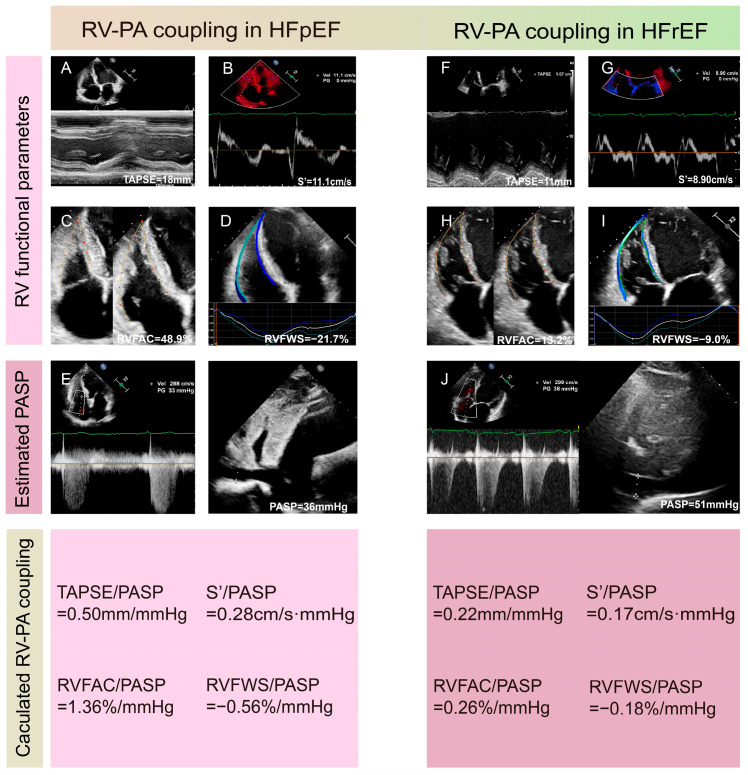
Examples of measurements of right ventricular-pulmonary artery coupling in HFpEF (A–E) and HFrEF (F–J) patients using 2DE and speckle tracking echocardiography. (A,F) Measurements of TAPSE. (B,G) Measurement of S’. (C,H) Calculation of RVFAC. (D,I) Evaluation of RVFWS. (E,J) Estimation of PASP using peak tricuspid regurgitation velocity, RVSP, and inferior vena cava diameter. 2DE: two-dimensional echocardiography; FAC: functional area change; HFpEF: heart failure with preserved ejection fraction; HFrEF: heart failure with reduced ejection fraction; PASP: pulmonary arterial systolic pressure; RVFWS: free wall longitudinal strain; RVSP: right ventricular systolic pressure; S’: tricuspid annular systolic velocity; TAPSE: tricuspid annular plane systolic excursion.
